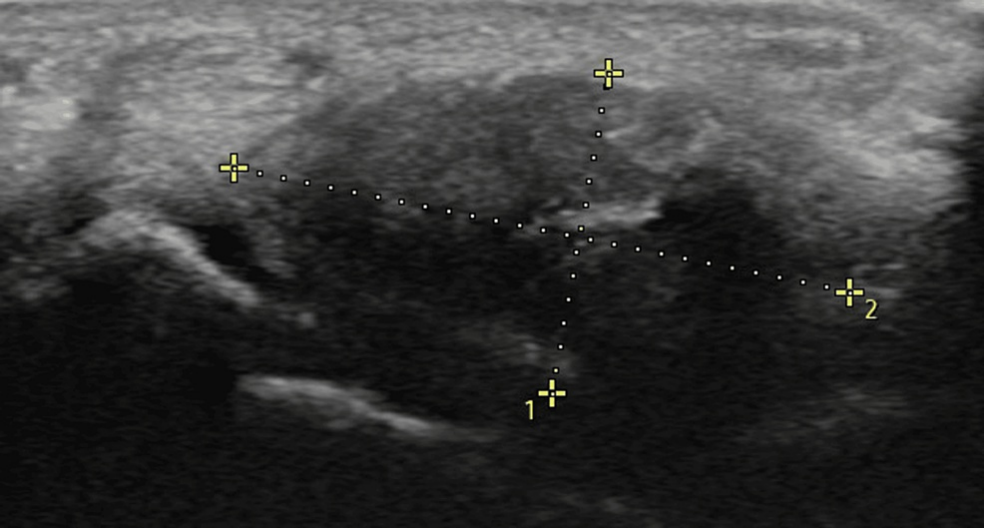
Ultrasound image of the measurement of the carpal tunnel 1. anteroposterior diameter; 2. transverse diameter
